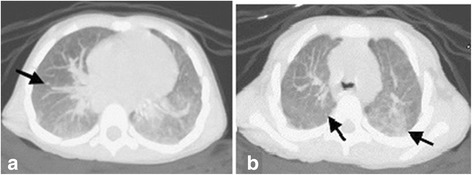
Pulmonary haemorrhage associated with idiopathic pulmonary haemosiderosis. a Axial computed tomography (CT) scan at the level of the lower lobes of the thorax (pulmonary window). b Axial CT scan at the level of the upper lobes of the thorax (pulmonary window). The images show diffuse ground-glass opacity associated with fine reticulation, findings characteristic of pulmonary haemorrhage. The predominant findings in the acute phase of idiopathic pulmonary haemosiderosis also include diffuse nodules (*black arrows*)
